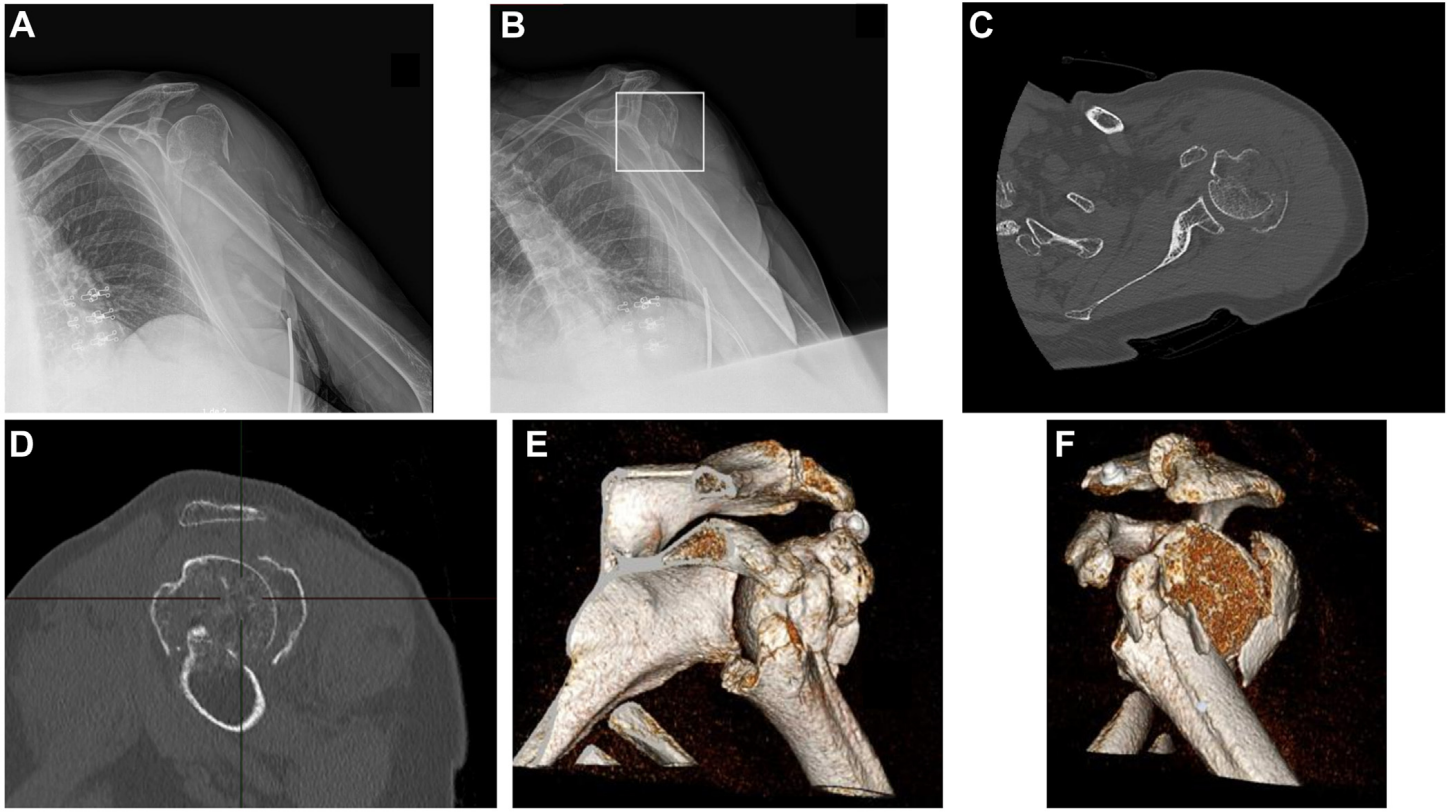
A 3-part fracture of the proximal humerus with a posteromedially displaced greater tuberosity evaluated through the radiographs (A and B), the CT axial (C) and sagittal (D) plane and 3-dimensional CT reconstruction (E and F). *CT*, computed tomography.
